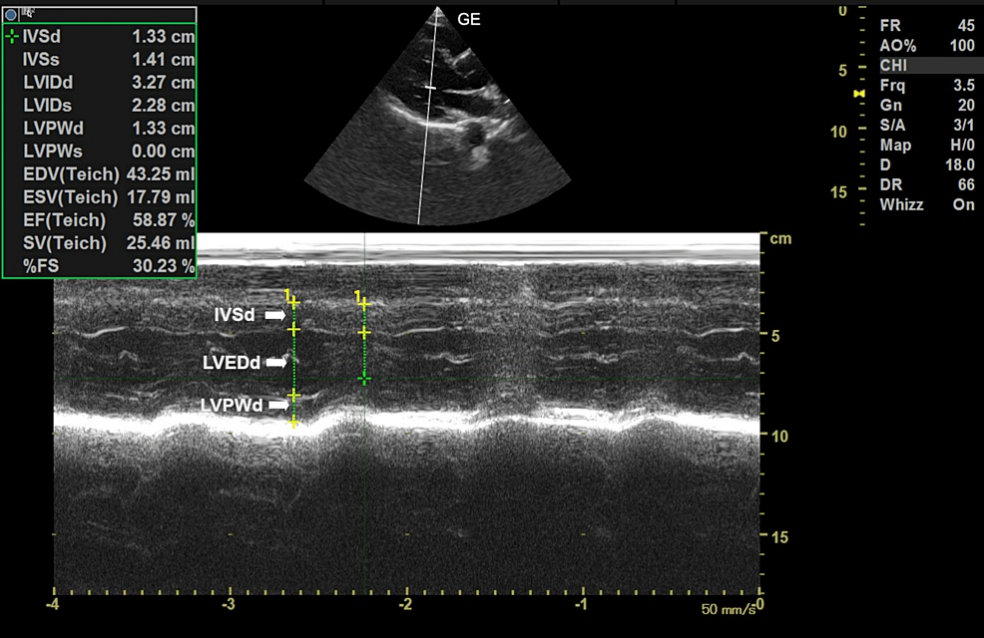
M mode in parasternal long axis view of transthoracic echo showing measurement of various parameters of left ventricular mass LVEDd: LV end diastolic diameter; IVSd: intraventricular septal diameter; LVPWd: LV posterior wall diastolic thickness
